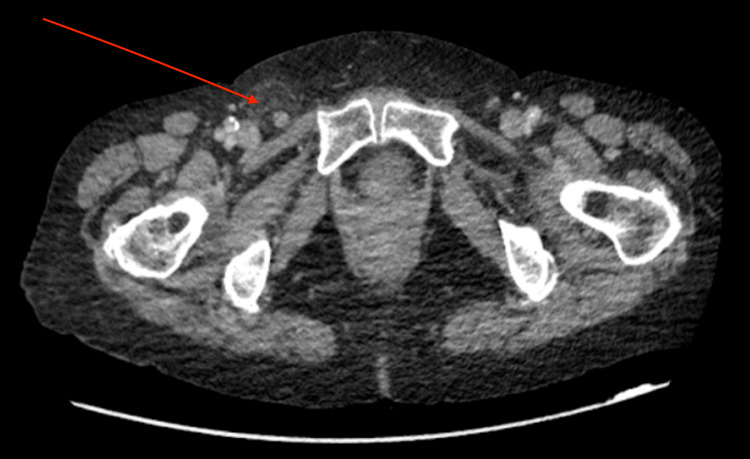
An axial section of a CT scan showing an inflamed appendix within a right-sided femoral hernia, indicated by an arrow
